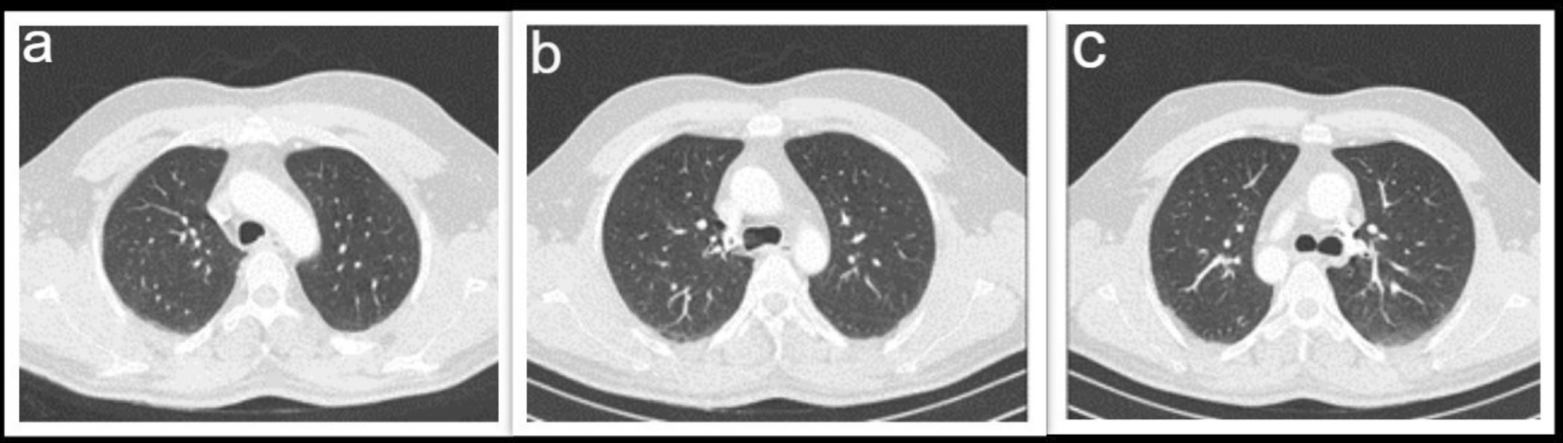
CT scan after 12 month follow up (a) mid portion of trachea, (b) distal part of trachea above the carina, (c) trachea in the level of carina.
